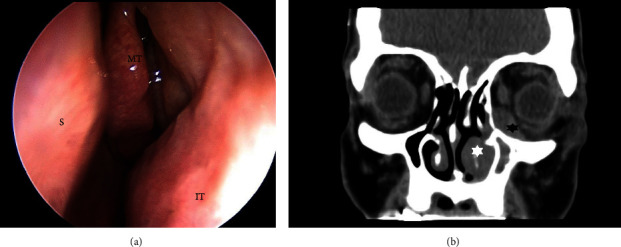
(a) Endoscopic image of the left nasal cavity that revealed the presence of pathologic tissue arising from the inferior turbinate and extending in the lateral nasal wall. (S: septum, MT: middle turbinate, and IT: inferior turbinate). (b) CT scan coronal view showing the presence of hypertrophic tissue of the lateral nasal wall and inferior turbinate (white star) extending inside the orbit (black star).
